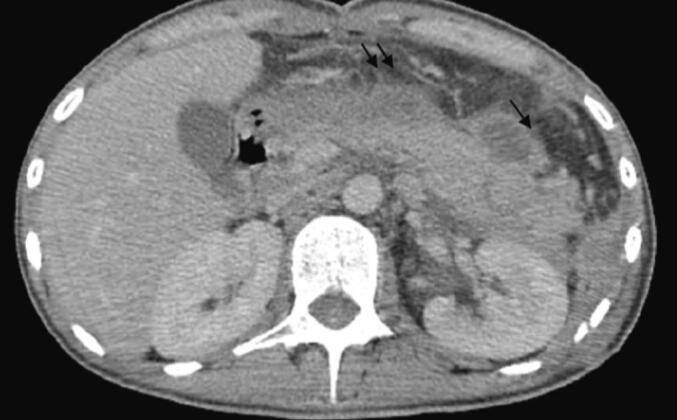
Axial CT section showing acute post-traumatic pancreatitis: peri-pancreatic necrosis and infiltration of peri-pancreatic fat.
